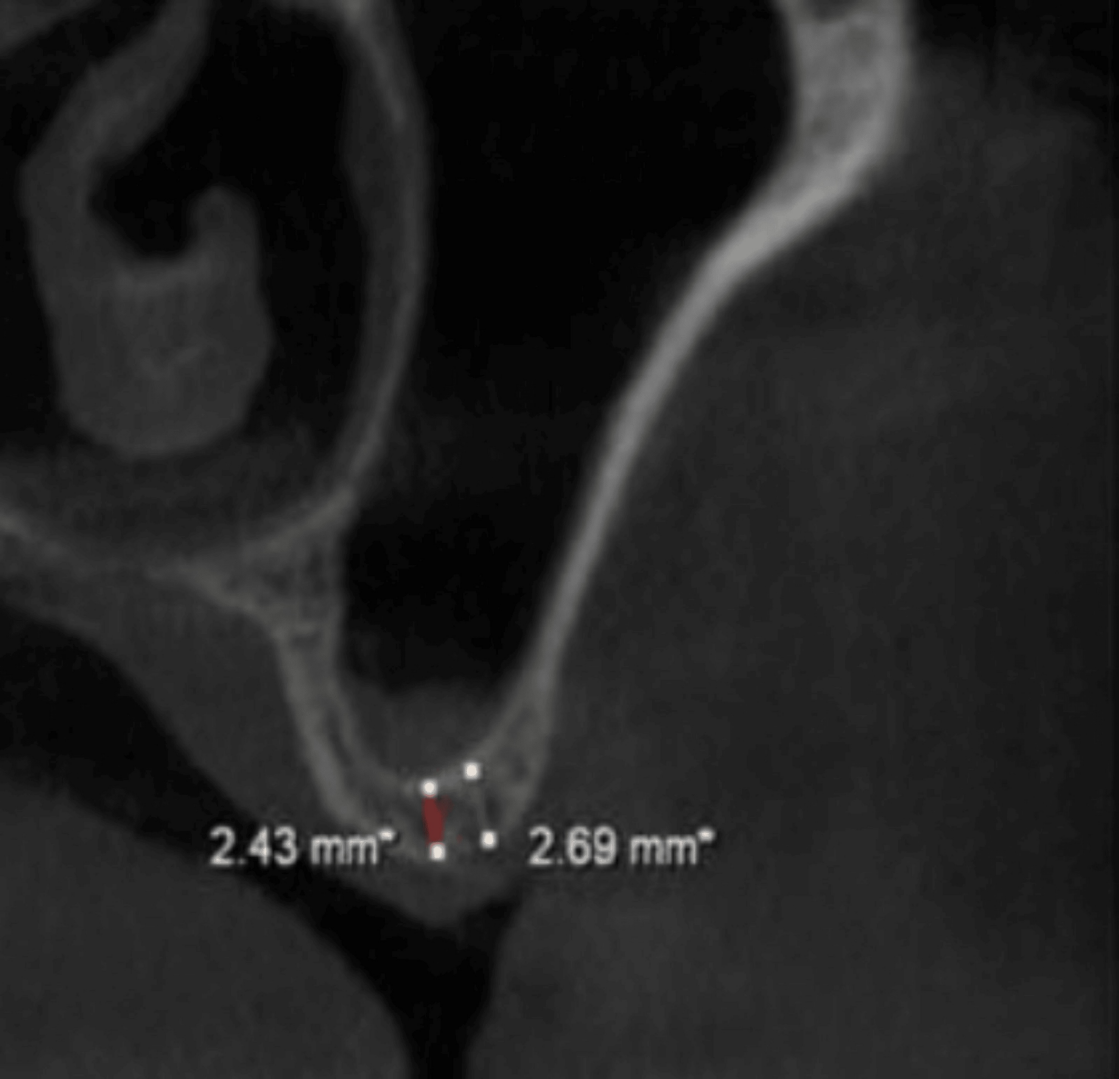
Preoperative CBCT depicting 2.43 mm residual ridge height under the sinus floor. CBCT: cone-beam computed tomography
